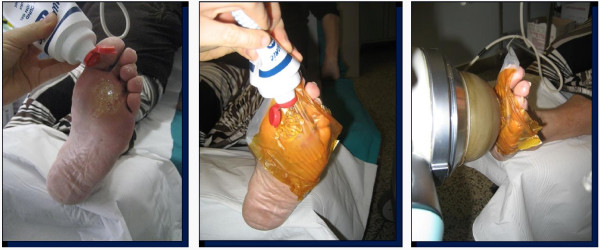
The steps of ultrasonic gel, plastic draping and shock wave administration at work in the study.
